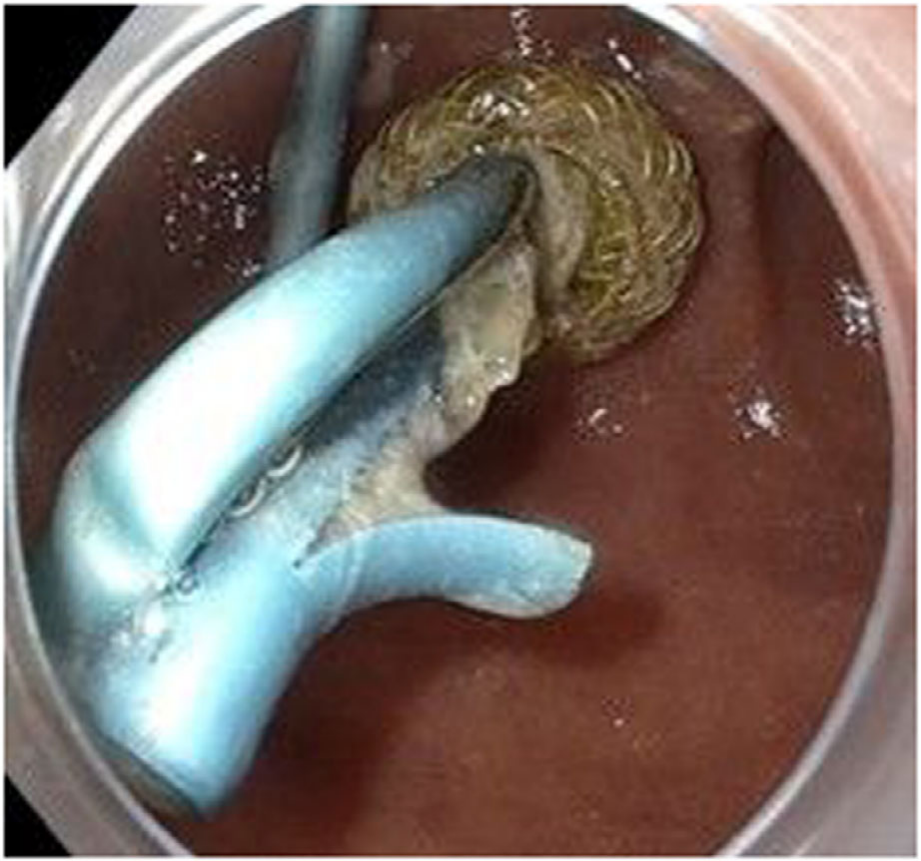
Occluded lumen‐apposing metal stent with plastic stents after endosonography‐guided choledochoduodenostomy.
